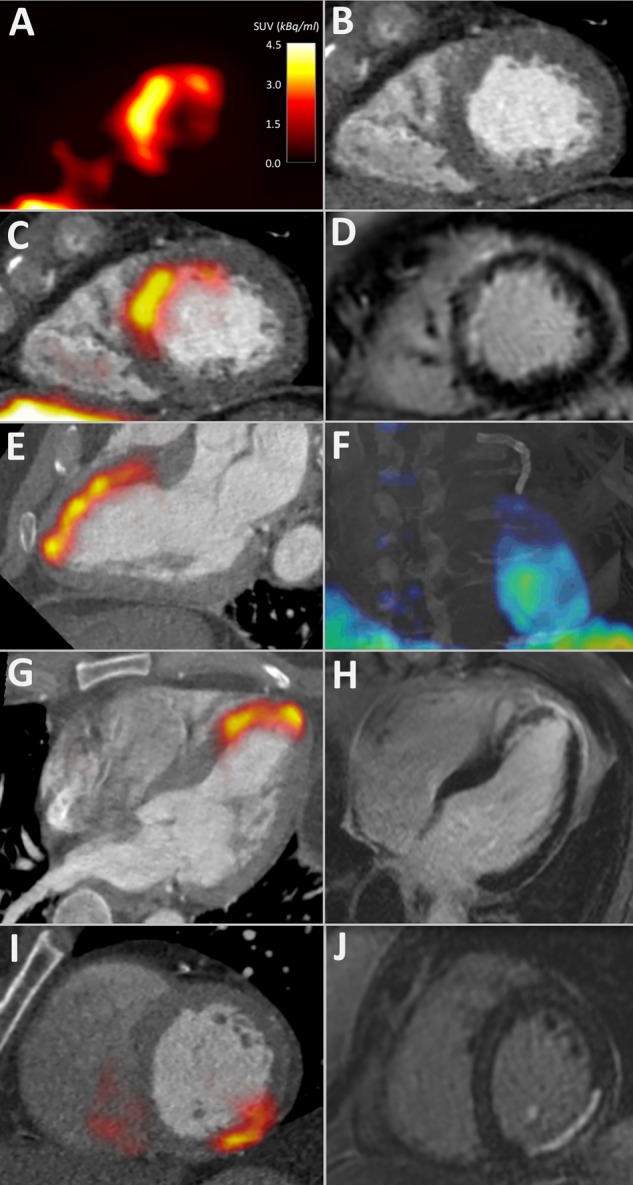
^18^F-Fluciclatide uptake in acute myocardial infarction (MI) is shown. 18F-Fluciclatide uptake in three patients with recent subendocardial MI is shown. Patient 1, 13 days after anterior MI, displaying a short-axis positron emission tomography (PET) image of the left ventricle with crescentic 18F-fluciclatide uptake (A) that correlates with the interventricular septum and anterior wall on CT angiography (B). The fused PET/CT-angiography image (C) shows this uptake to correspond exactly with the region of late gadolinium enhancement (LGE) on cardiac magnetic resonance (CMR) (D). Further delineation of myocardial uptake on PET/CT is clearer in the two-chamber view (E) and on a fused CT/three-dimensional-Patlak image, which shows this uptake to follow a watershed-pattern emerging from the coronary stents present in the left anterior descending coronary artery (F) (see online supplementary video file 1). (G) and (H) Patient 2, 8 days following anterior MI, displaying focal uptake of 18F-fluciclatide in the anterior wall and apex in the three-chamber view on PET/CT (G) which corresponds to the region of infarction on LGE CMR imaging (H). (I) and (J) Patient 3, showing focal uptake of 18F-fluciclatide in the inferior wall 19 days following MI on PET/CT (I) that again corresponds to the infarction on CMR LGE imaging (J).
